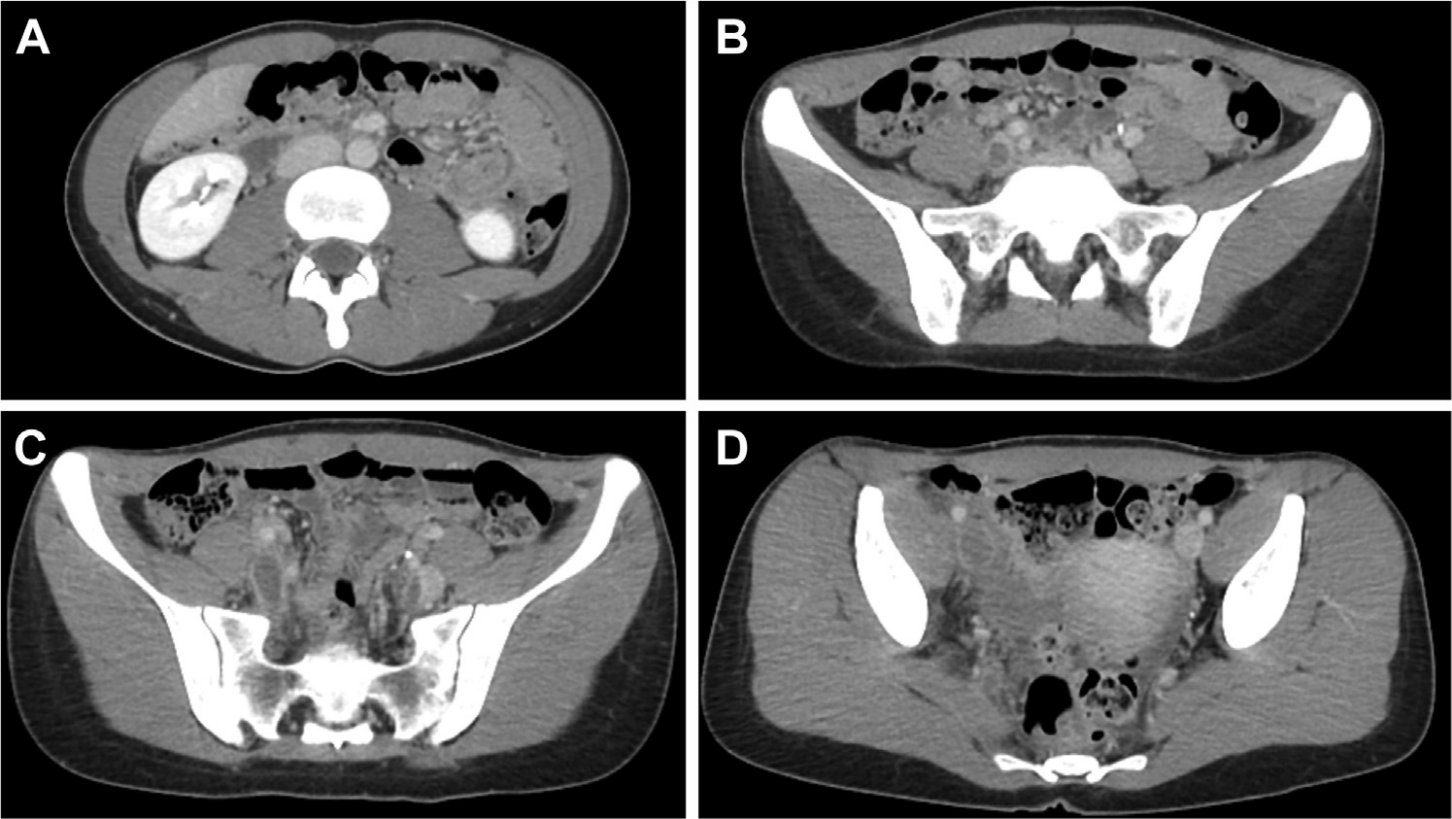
Computed tomography venogram demonstrating no thrombus in the inferior vena cava (A), occlusive thrombus within bilateral internal iliac veins (B and C), partial occlusion of the left common iliac vein due to propagation of the internal iliac thrombus, and complete occlusion of the right common iliac and external iliac veins (C and D).
